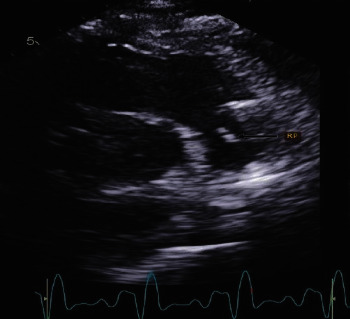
Echocardiographic view of the Impella RP in the right ventricular outflow tract.
